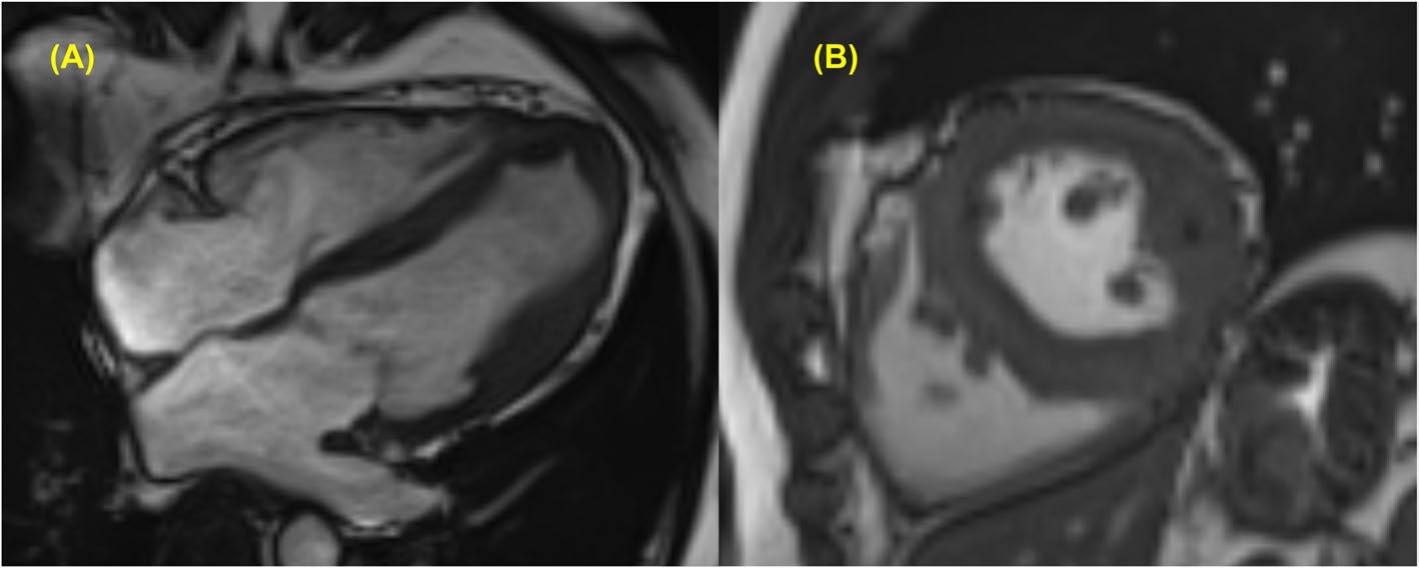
Cardiac magnetic resonance images showing atypical presentations of hypertrophic cardiomyopathy. Hypertrophied myocardium of the mid-anterolateral wall is observed in both (A) the apical four-chamber view and B the parasternal short-axis view
